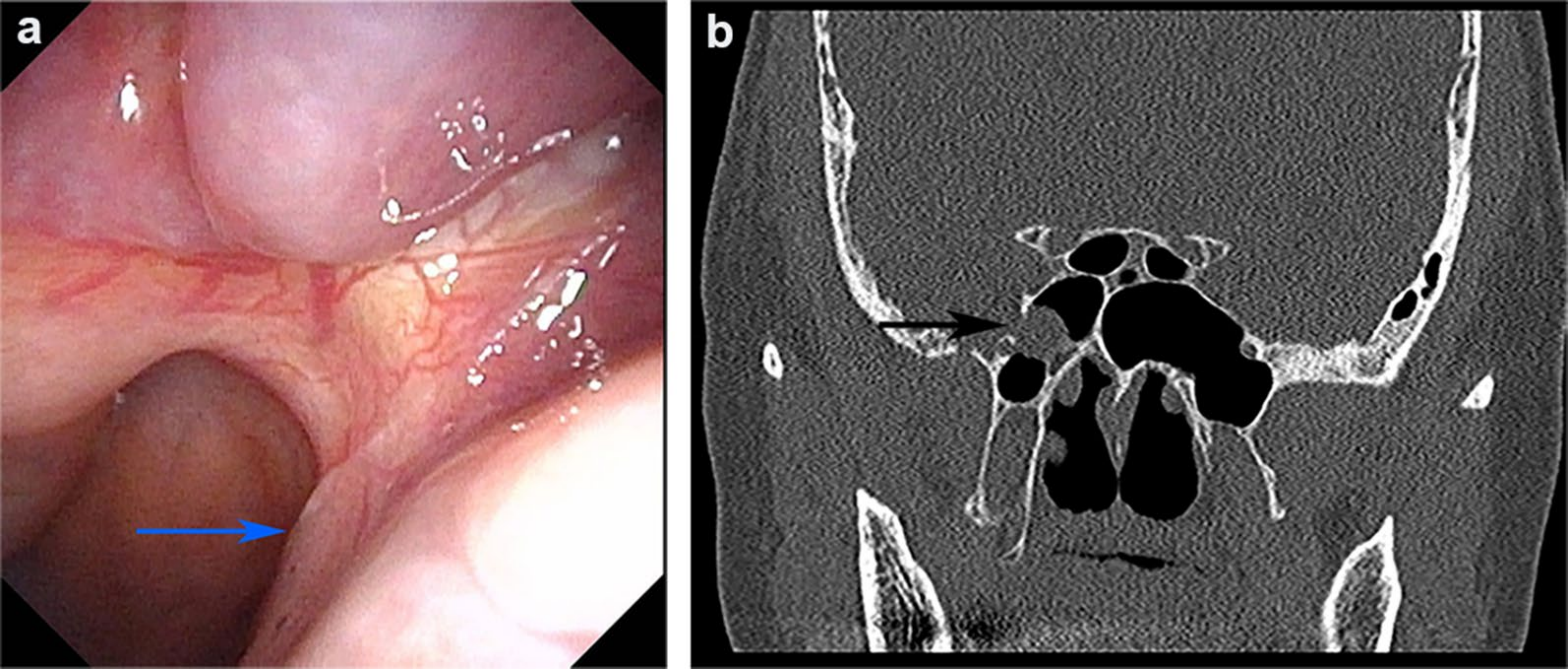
Preoperative endoscopy image and sinus computed tomography (CT) scan. a Preoperative endoscopy image. The blue arrow indicates the persistent flow of cerebrospinal fluid (CSF) backwards into the right posterior nostril. b Sinus CT scan. The black arrow indicates the place of lesion tissue protruding into right sphenoid sinus
